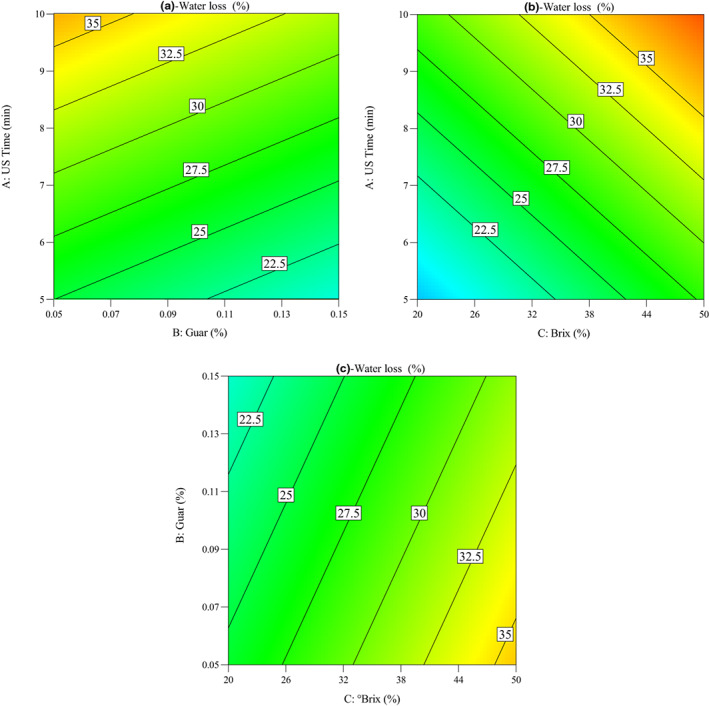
Contour plots for the impacts of operating conditions on the water loss of quince slices: (a) ultrasound time versus guar concentration, (b) ultrasound time versus °Brix, and (c) guar concentration versus °Brix.
